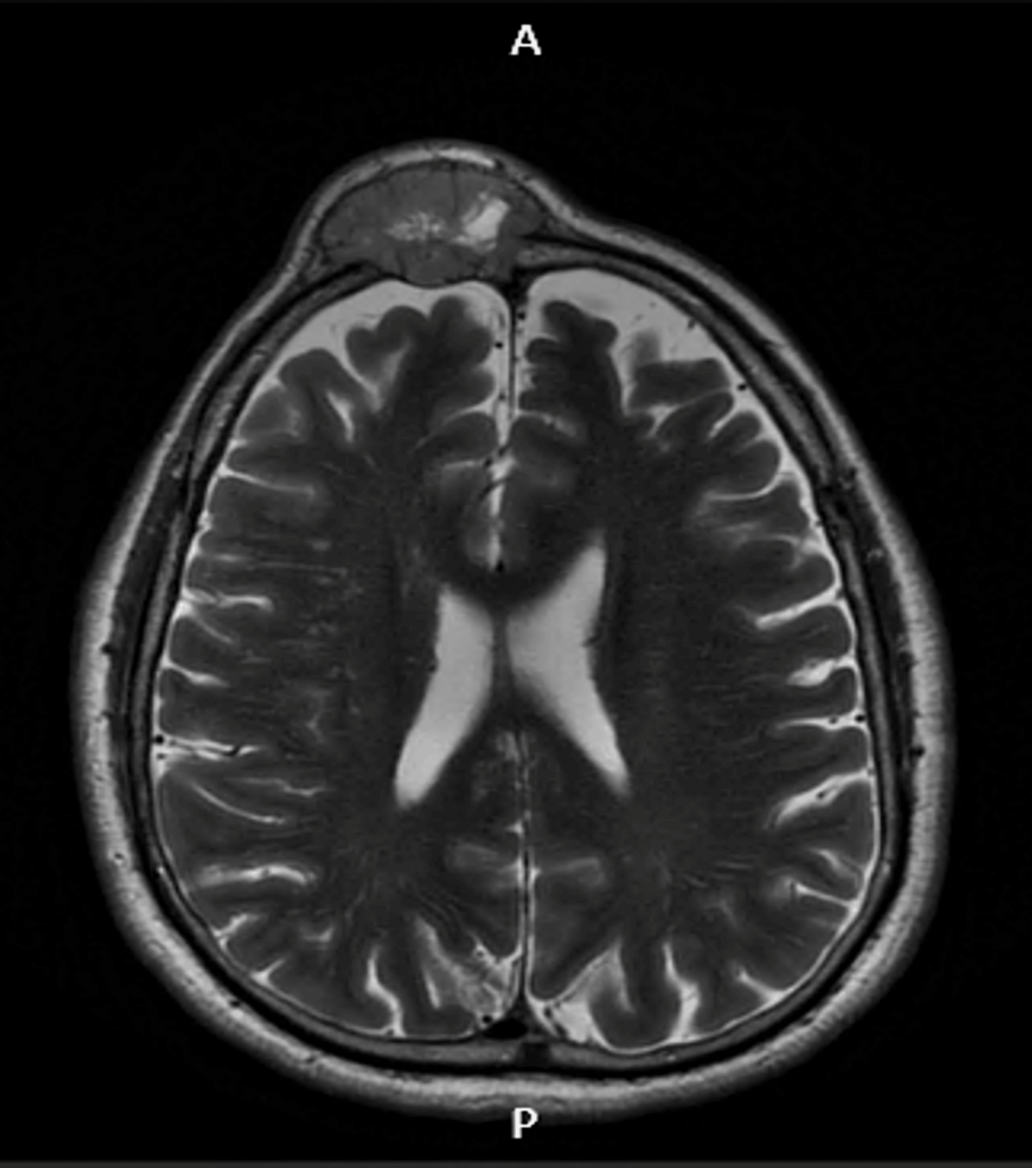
Cranioencephalic MRI in axial section The image shows an axial section in T1 fluid-attenuated inversion recovery (FLAIR).
